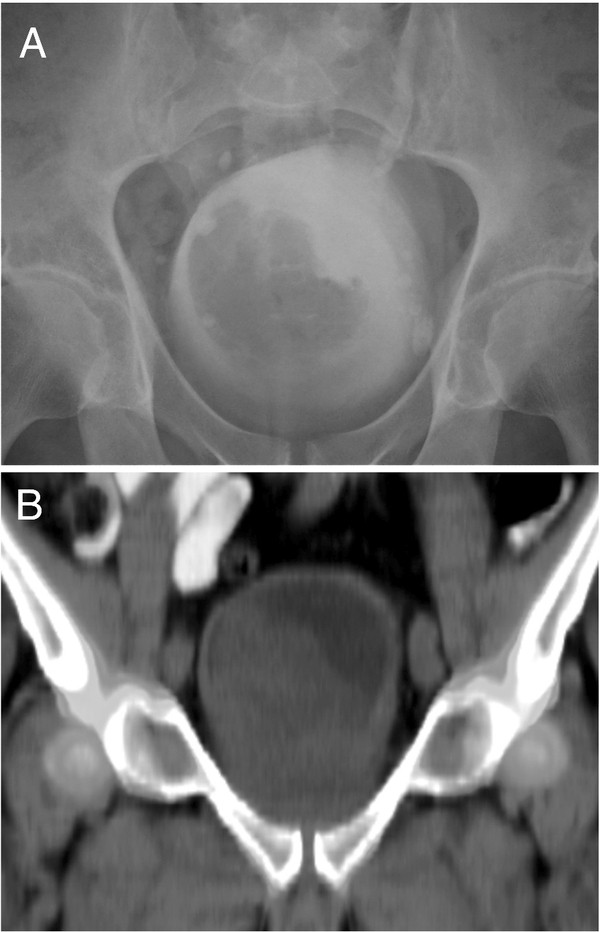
IVU and CT Images Demonstrating the Intravesical Projection of the Giant Benign Prostate with Nephrogenic Adenoma. Image A - Intravenous Urogram from August 2007. Image B - Volumetrically reconstructed CT image from October 2007.
